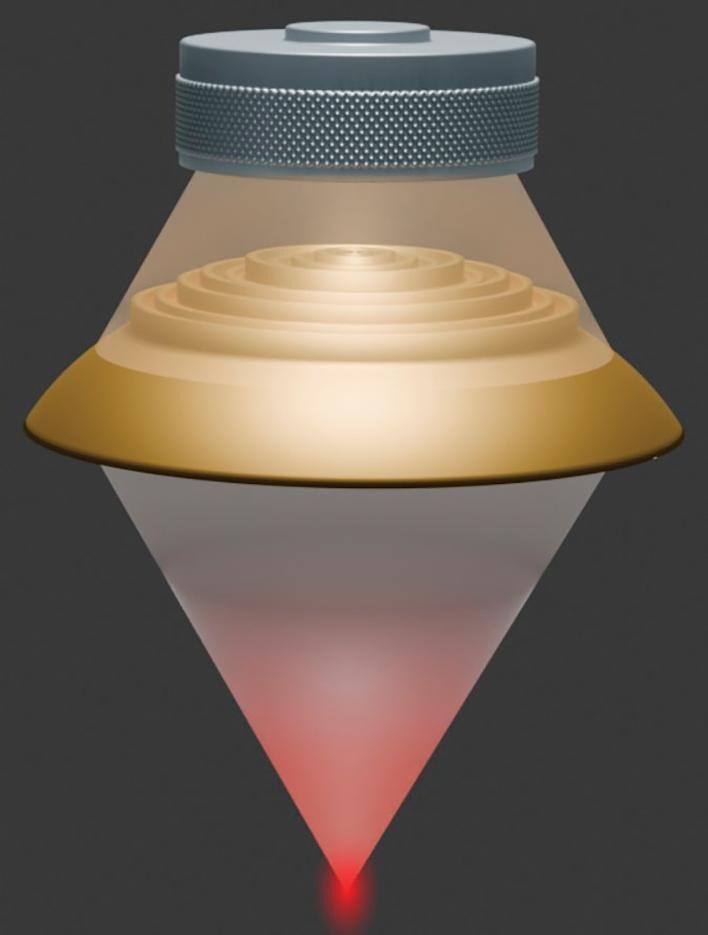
An inversely designed metalens, optimized using a genetic algorithm, can significantly enhance ultrasound transmission through skull-like rigid materials while providing focusing function (*Courtesy of Prof. Jie Zhu*).
